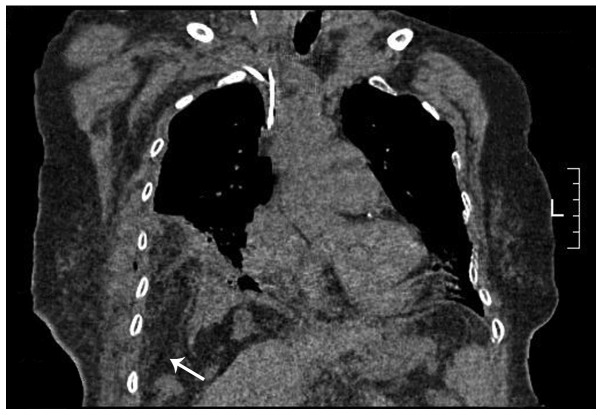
A coronal thoracic CT image shows a right diaphragmatic defect and loops of the bowel protruding into the thoracic cavity, as indicated by the white arrow. CT, computed tomography.
